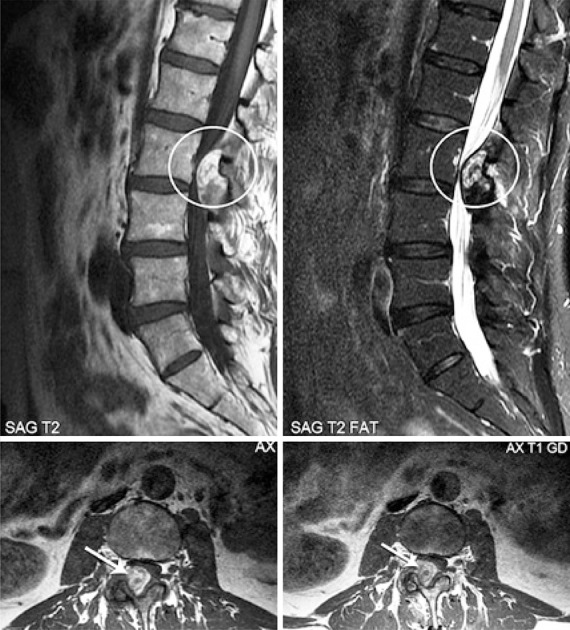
Magnetic resonance imaging showing heterogeneous intraspinal and extradural mass, contiguous to the interapophyseal joint at L2-L3, on the right, with radicular and dural compression, with no signs of fat. Parietal enhancement after contrast
